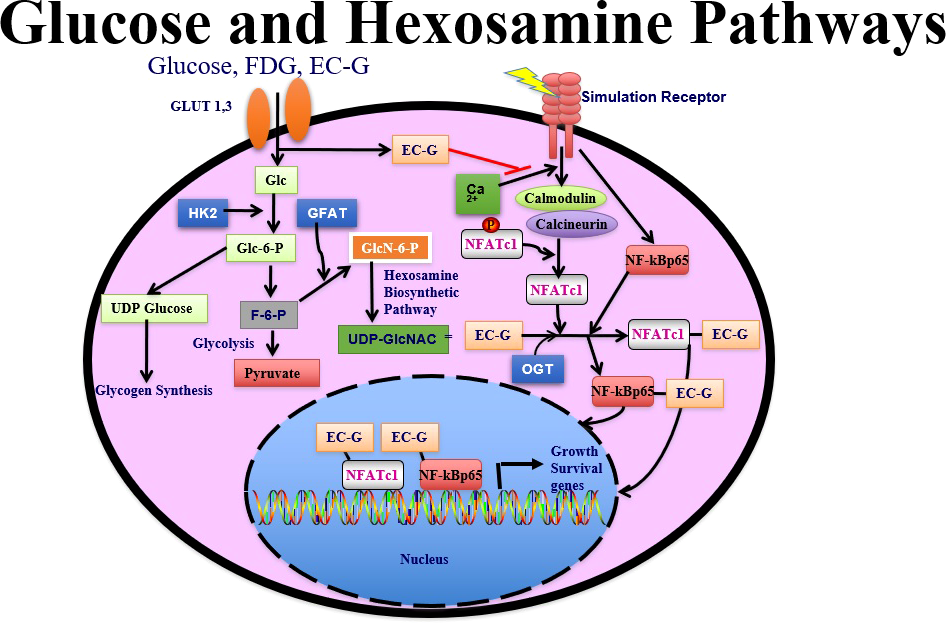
FDG is involved in glycolysis. EC-G has downstream to OGT glycosylation pathways. ^99m^Tc-EC-G measures cell cycle progression in cancer.
